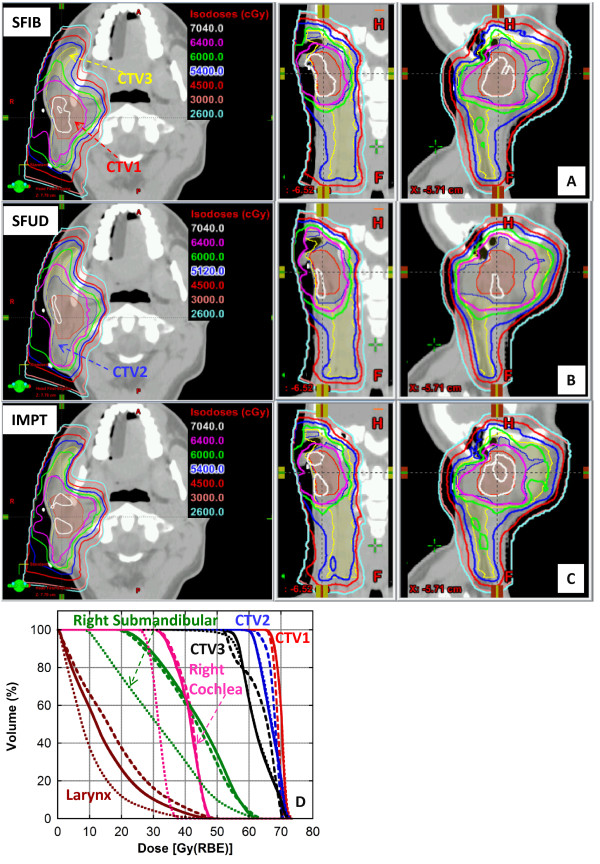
Representative axial, coronal and sagittal (left, middle, and right panels, respectively) CT images for a patient 4. (A) SFIB, (B) SFUD with sequential boost and (C) IMPT treatment plans. Targets volumes are represented as color washes. (D) Dose volume histogram (solid lines, SFIB; dashed lines, SFUD and dotted line, IMPT).
